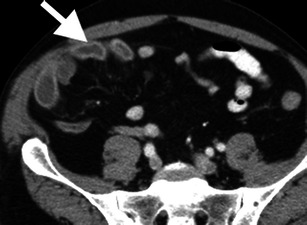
Hyper-enhancement of the bowel mucosa. Axial contrast-enhanced CT image demonstrates hyper-enhancement of the right lower quadrant ileal mucosa (*arrow*) secondary to superior mesenteric artery occlusion (not shown). The degree of mucosal enhancement can be compared to left-sided bowel loops as an internal control
